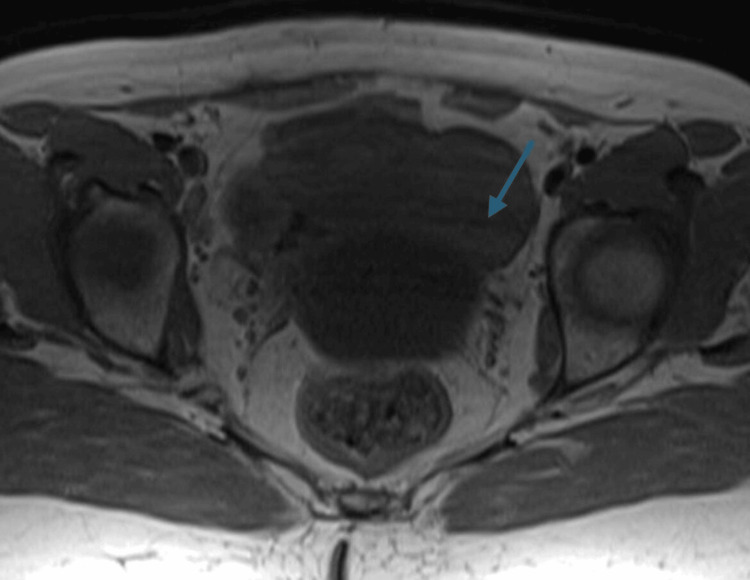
Lipoleiomyoma seen in Figure 2 shows low intensity following fat-suppressed imaging on MRI, confirming the adipocytic component (see arrowhead).
